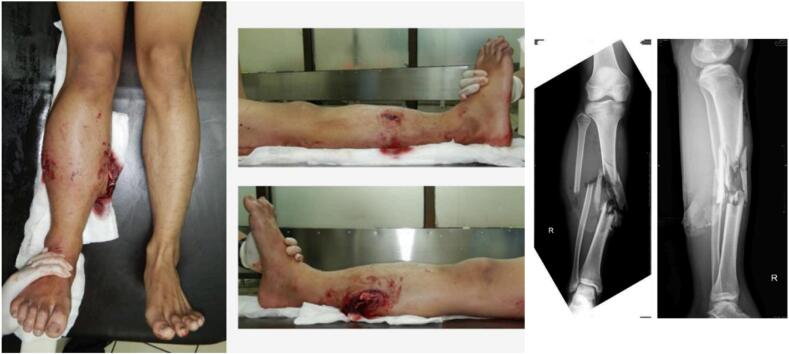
Degree of exposure of the fracture and soft tissue at the level of the right leg. (left and middle). Pre-surgical emergency radiographs (right).
